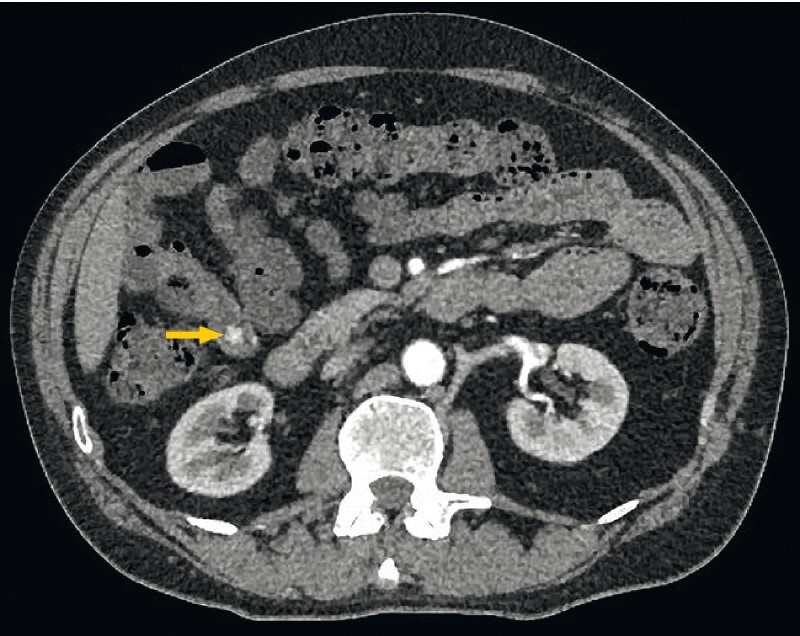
Abdominal computed tomography scan showing a polypoid lesion within the distal small bowel with hyperenhancement on the arterial phase.
